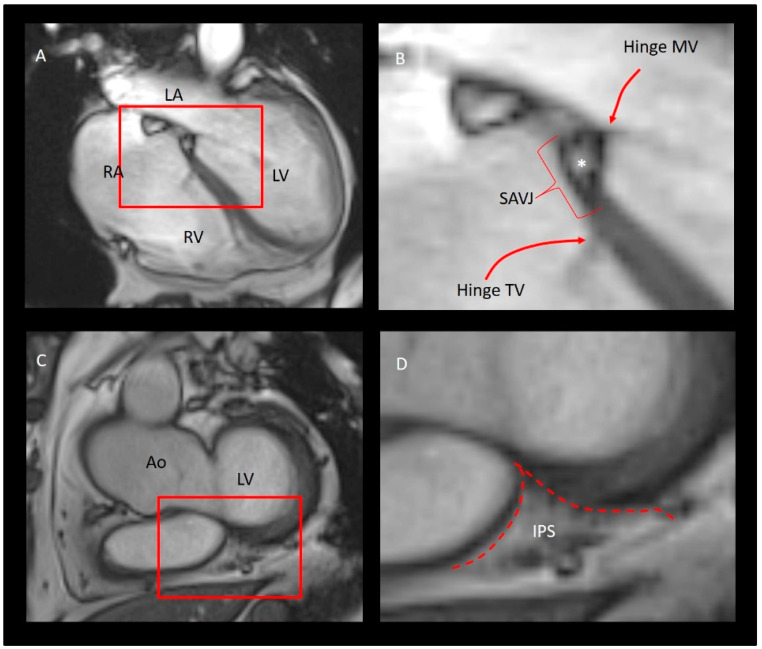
(A). CMR four chamber view. (B) magnified image of the structure inside the read box. The image shows the septal atrioventricular junction (SAVJ), the hinge line of tricuspid (TV), and mitral (MV) valves. The asterisk points at the leaf of adipose tissue in between the right atrial wall on the right side, the crest of the interventricular septum on left side (see text). (C) MRI short-axis view on the base of left ventricle (LV). (D) Magnified image showing the inferior pyramidal space (IPS). The red dotted line marks the boundaries of the IPS. RA = right atrium; LA = left atrium; RV = right ventricle; LV = left ventricle; Ao = aorta.
